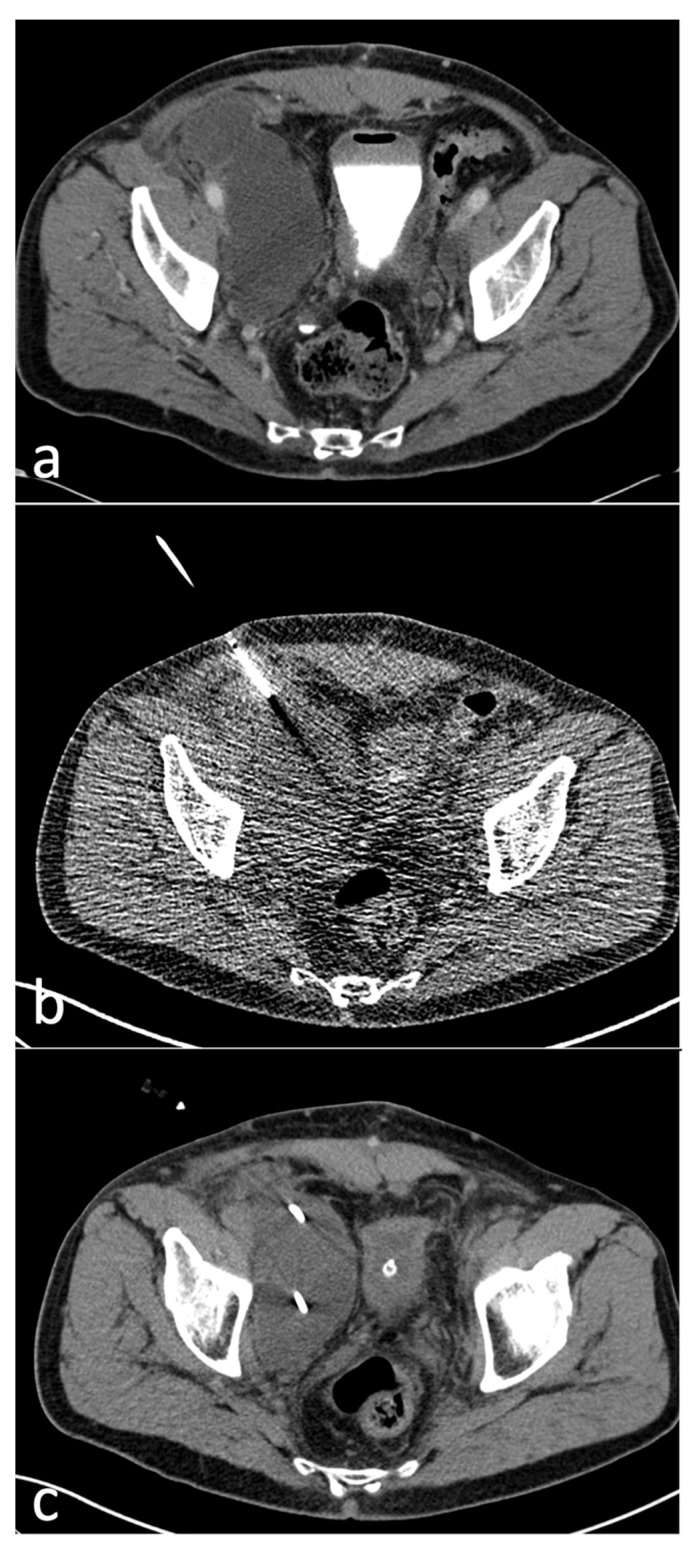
(a)-Axial CT image showing a right-sided Type 2A lymphocele, 10 days after radical prostatectomy and pelvic lymph node dissection. The maximum diameter of the lymphocele (cranial extension not shown on this image) was 12 cm. (b)-Axial CTF image showing 8F-drain insertion with the trocar technique in the same Type 2A lymphocele using a tube current-time product of 10 mAs. (c)-Axial post-interventional CT image of the same patient showing successful 8F-drain insertion into the lymphocele.
